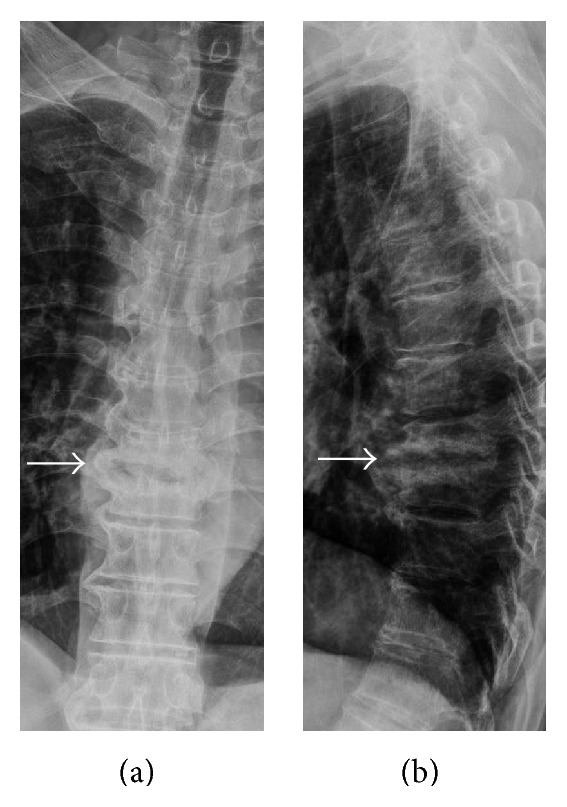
The plain films 6 months after operation. (a) AP view and (b) lateral view revealed prominent syndesmophyte formation at anterior and lateral aspects of infective area without significant kyphotic deformity.
